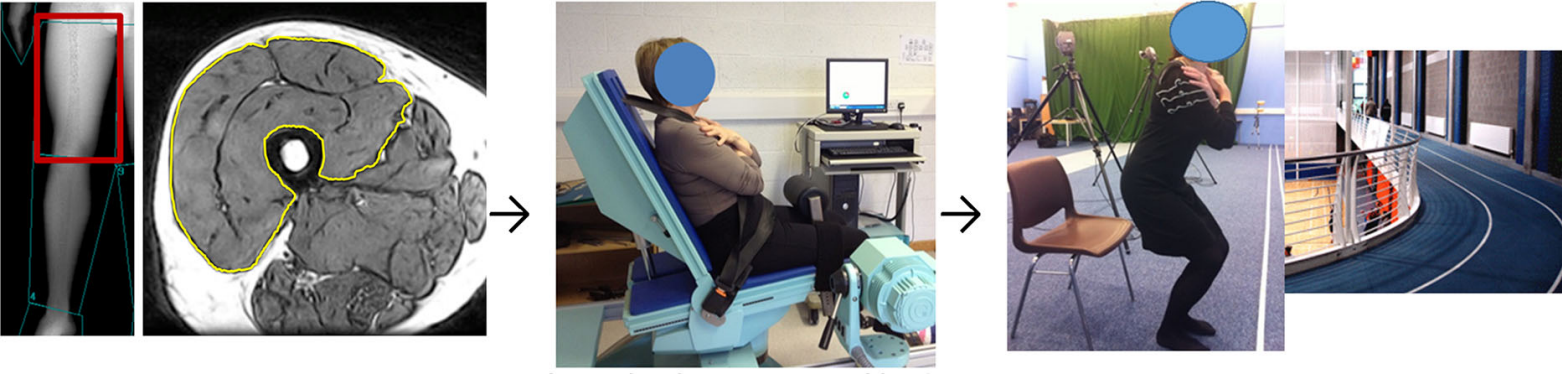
Upper leg lean tissue mass (iDXA; University of Limerick Body Composition Study) and quadriceps skeletal mass (MRI; Manchester Metropolitan Healthy Ageing Study), knee extensor torque (isokinetic dynamometry; University of Limerick Healthy Ageing Study), extended chair rise and gait speed tests (University of Limerick Healthy Ageing Study) appear to represent the indices of lower extremity muscle mass, strength and function responsive to age-related change and therapeutic intervention
